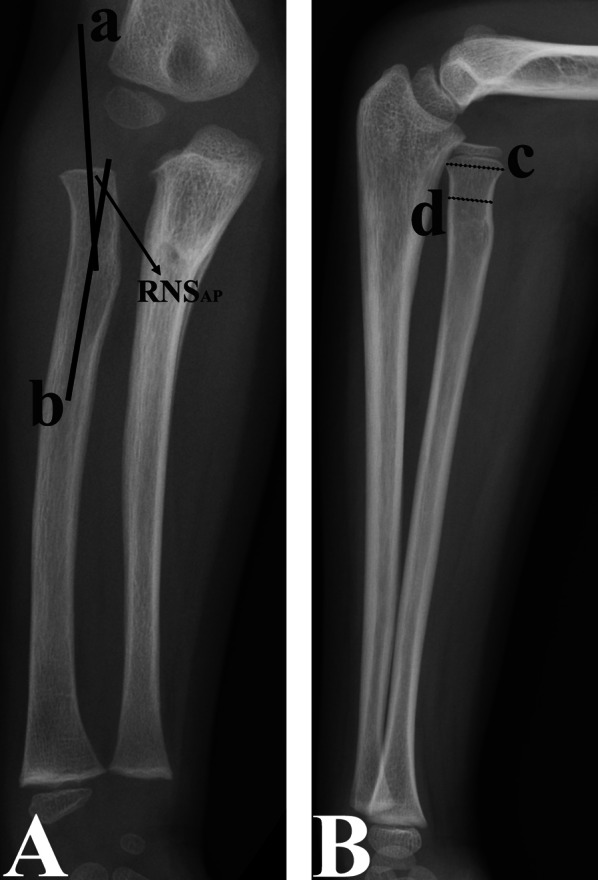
Measurement of the RNS_AP_ angle and RH_L_ size on anteroposterior (A) and lateral (B) radiographs. RNS_AP_ angle (marked with black arrow): the angle between line a (the radial neck axis) and line b (the proximal radial shaft axis) on the anteroposterior radiograph (A); RH_L_ size: the ratio of the width of the widest part of the proximal radial metaphysis (line c) to the narrowest radial neck width (line d) on the lateral radiograph (B)
